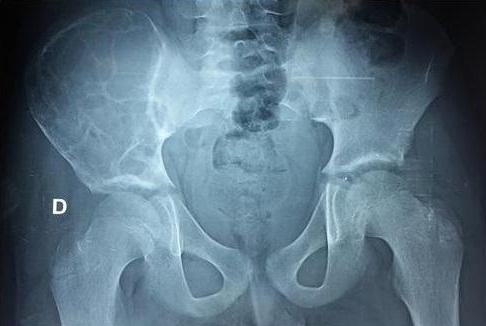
Radiographie standard du bassin de face montrant le plus souvent des images lytiques aréolaires mal limitées, réalisant l’aspect classique en « nid d’abeille ». Il n’existe ni réaction périostée ni décalcification régionale
